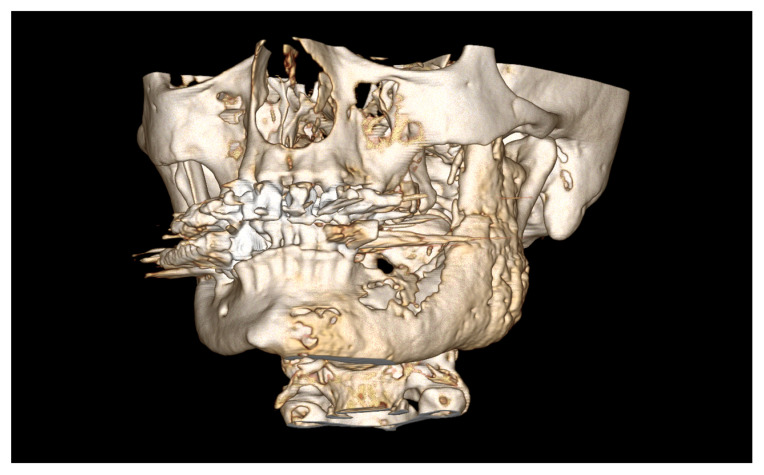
CT reconstruction of the midface and mandible shows a massive periost reaction due to MRONJ of the mandible.
